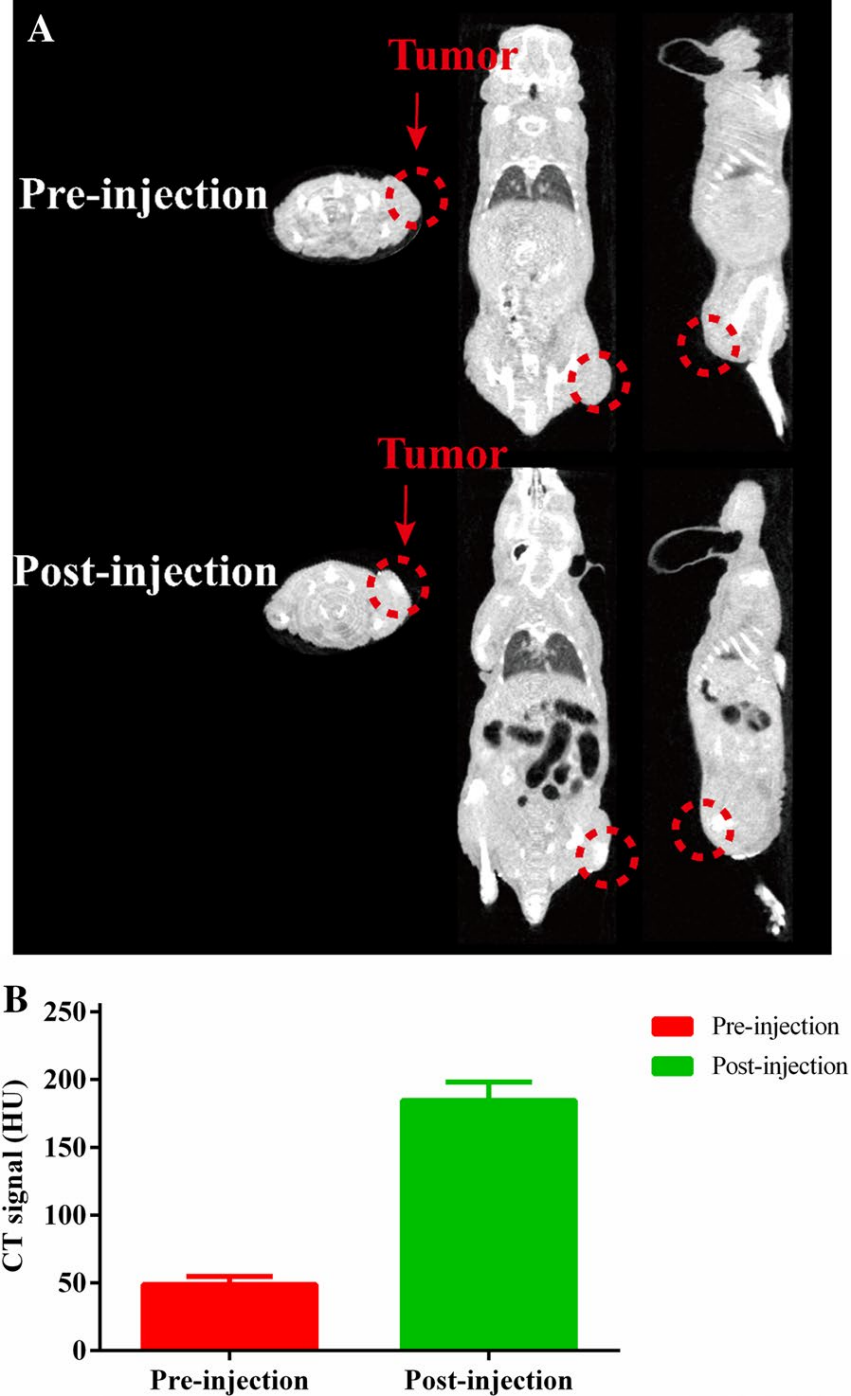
BiF_3:_ Ln@PVP NPs in vivo CT imaging effect. A CT images before and after BiF_3:_ Ln@PVP NPs injection and B the corresponding CT value. The red circle indicates tumor tissue
